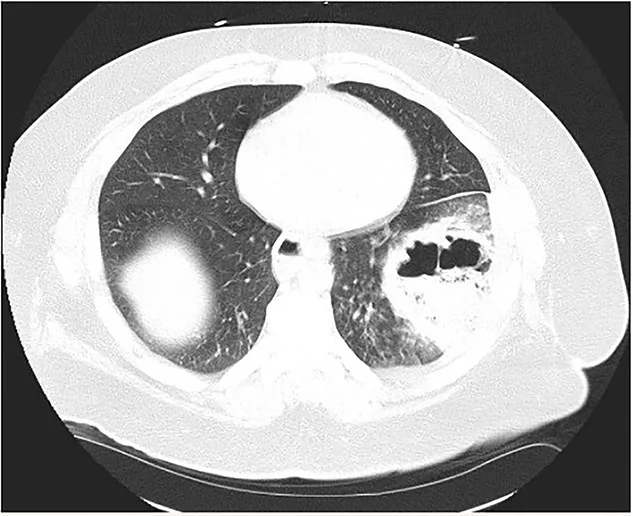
A CT scan showing a large lung abscess in the superior segment of the lower lobe of the left lung.
